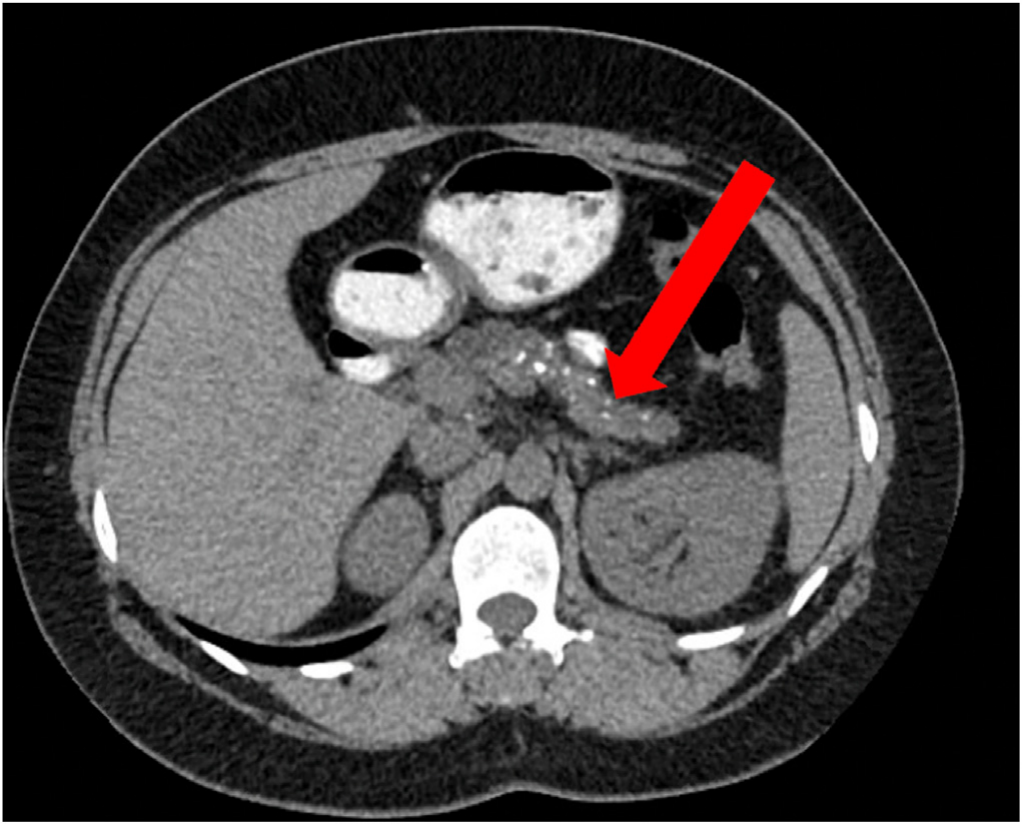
Computed tomography image showing an atrophic pancreas with multiple calcifications (*arrow*) consistent with chronic pancreatitis.
